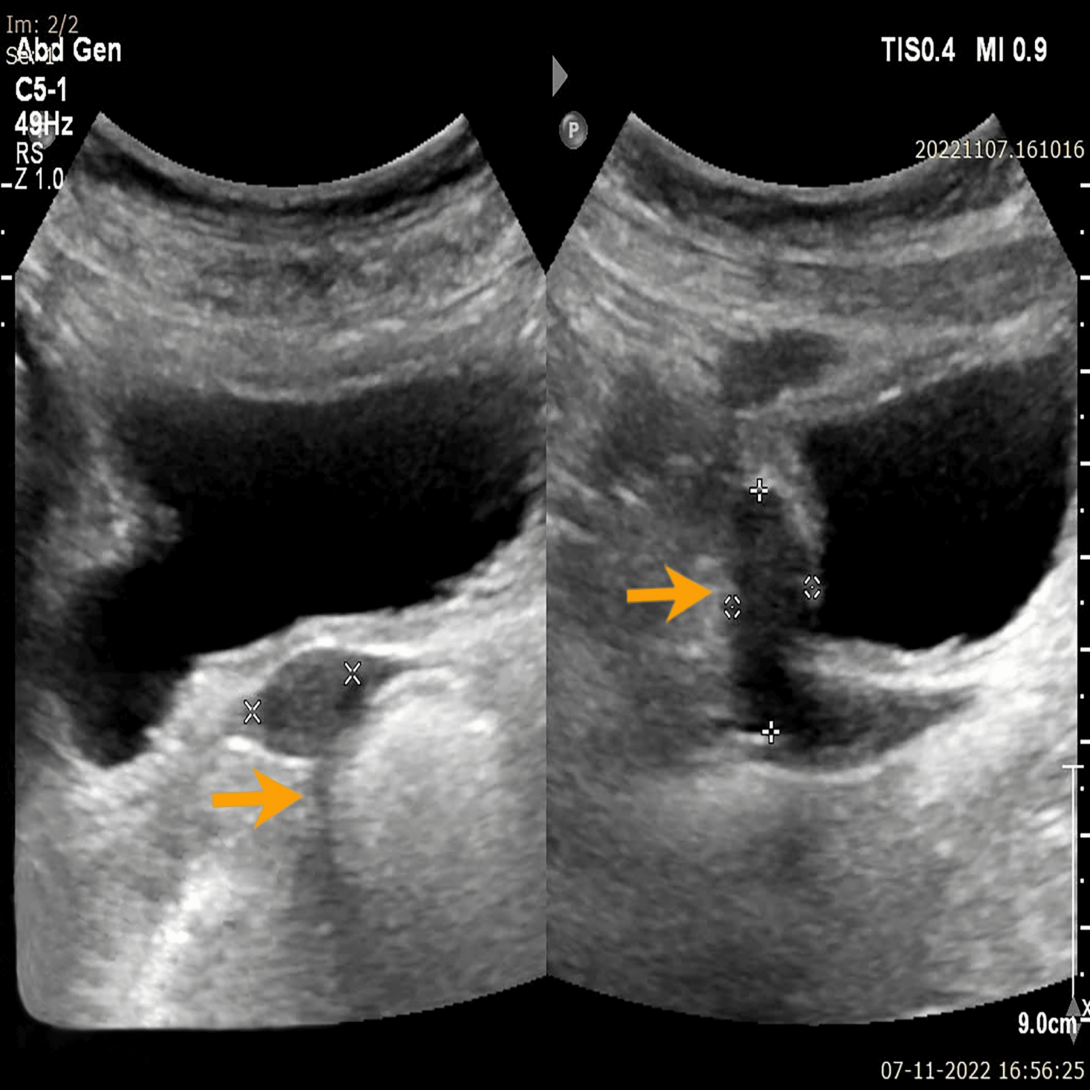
Ultrasound pelvis, showing hypoplastic uterus (orange arrows) with no visualized ovaries
